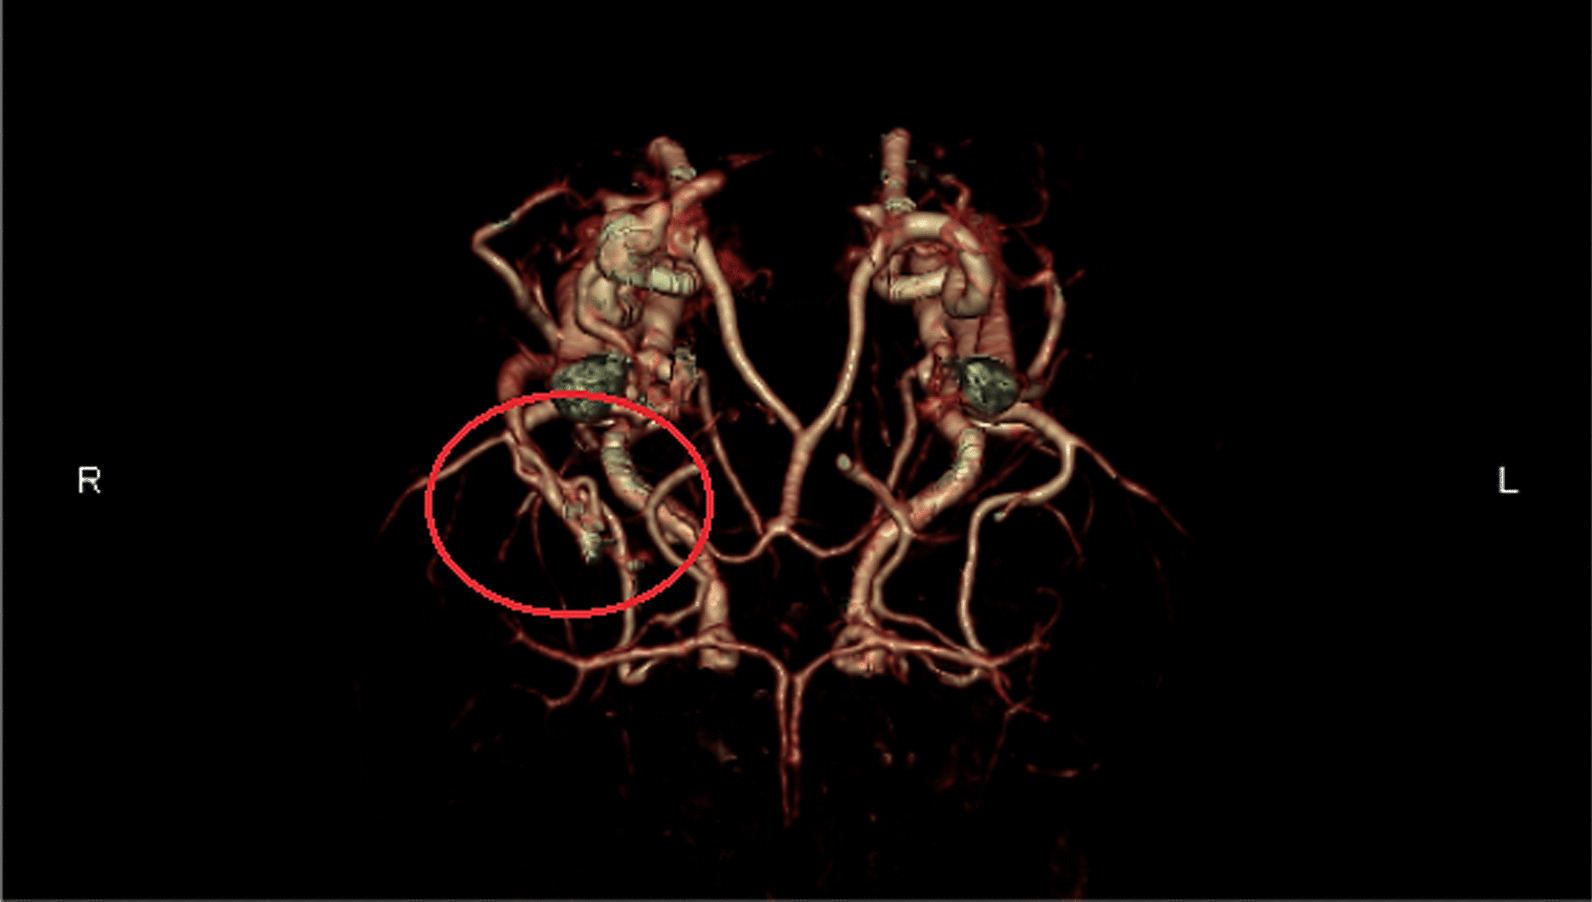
Contrast enhanced computed tomography angiography. Cranio-caudal 3D view showing vascular proliferation in the right side (red ring) comparing to the left side
